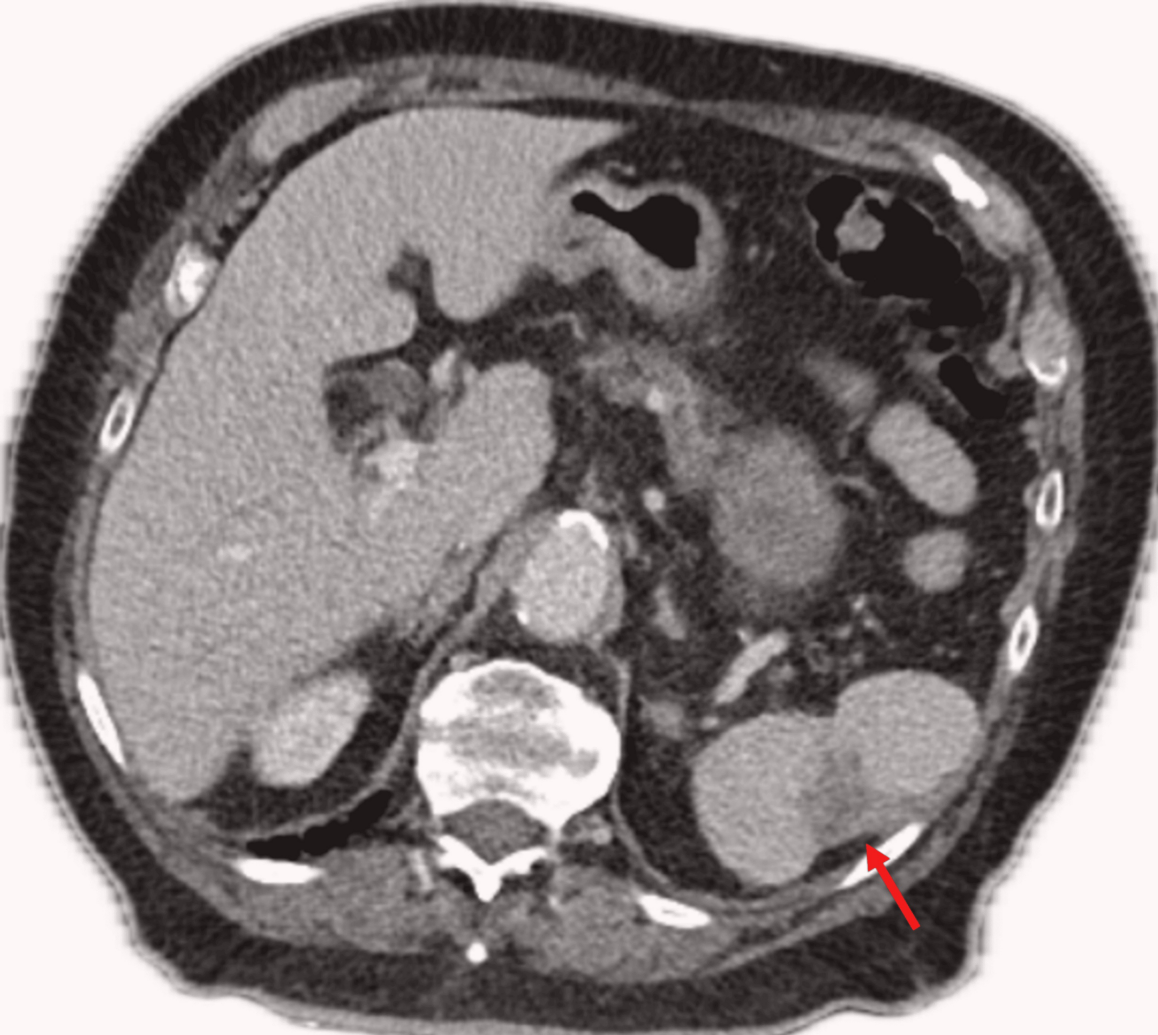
Stable CT appearance at two-month post presentation (red arrow)
